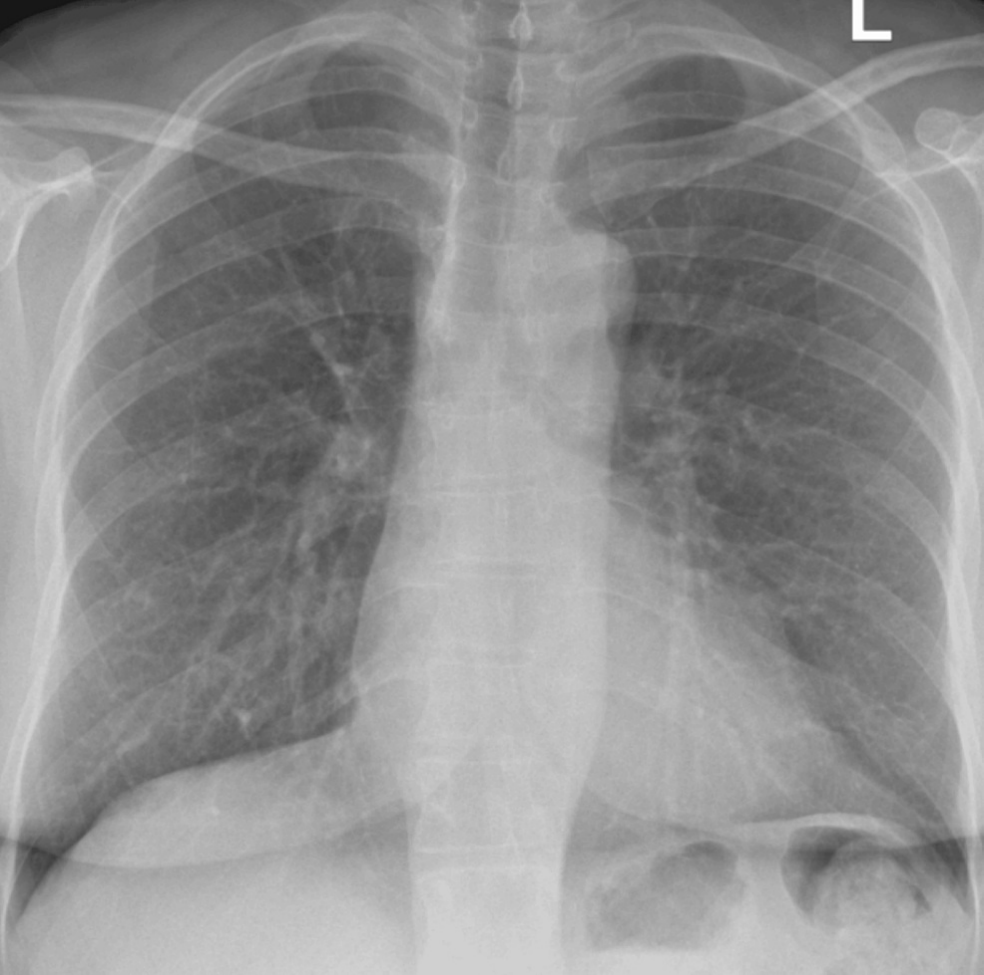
Chest X-ray taken during the first outpatient follow-up visit one month after discharge. The image shows significant improvement when compared to the chest X-ray from four weeks ago.
